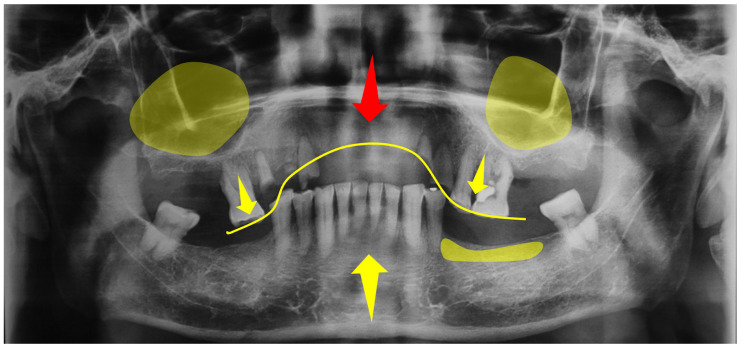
Orthopantomography that highlights areas potentially signaling bone loss. Yellow arrows are used to pinpoint specific instances of tooth extrusion. The red arrow points to the maxillary area, which represents bone loss and atrophy. Additionally, a yellow line traces the curve of Spee, highlighting the natural curvature of the biting surfaces of the teeth and providing insight into the alignment and bite relationship between the upper and lower dental arches. The X-ray image is critical for diagnostics for dental professionals in assessing the overall oral health of a patient, aiding in treatment planning, and monitoring dental and skeletal development. Source: the authors.
